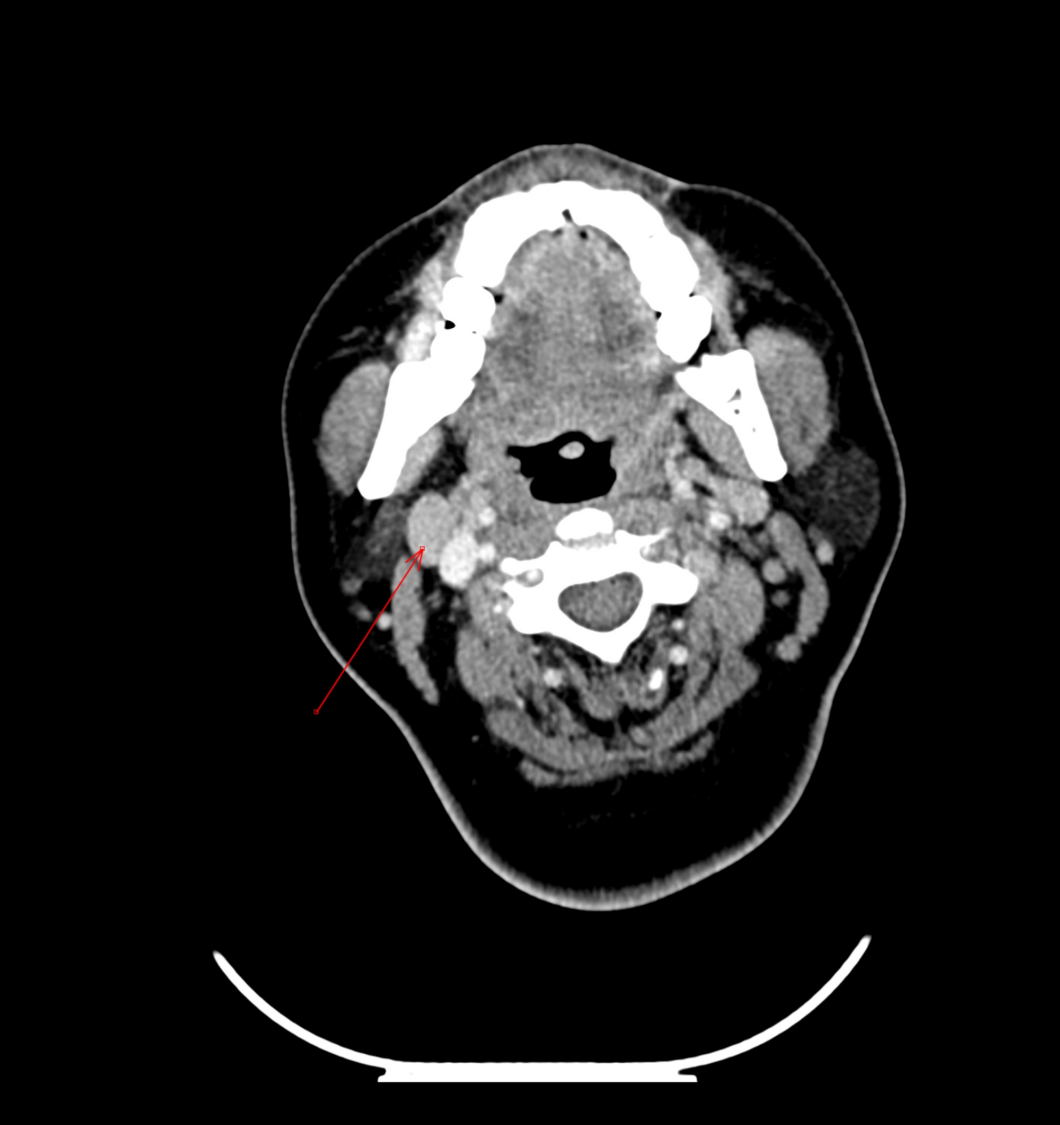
CT scan of the neck, arrow shows an enlarged cervical lymph node, anterior to the right jugular vein.
